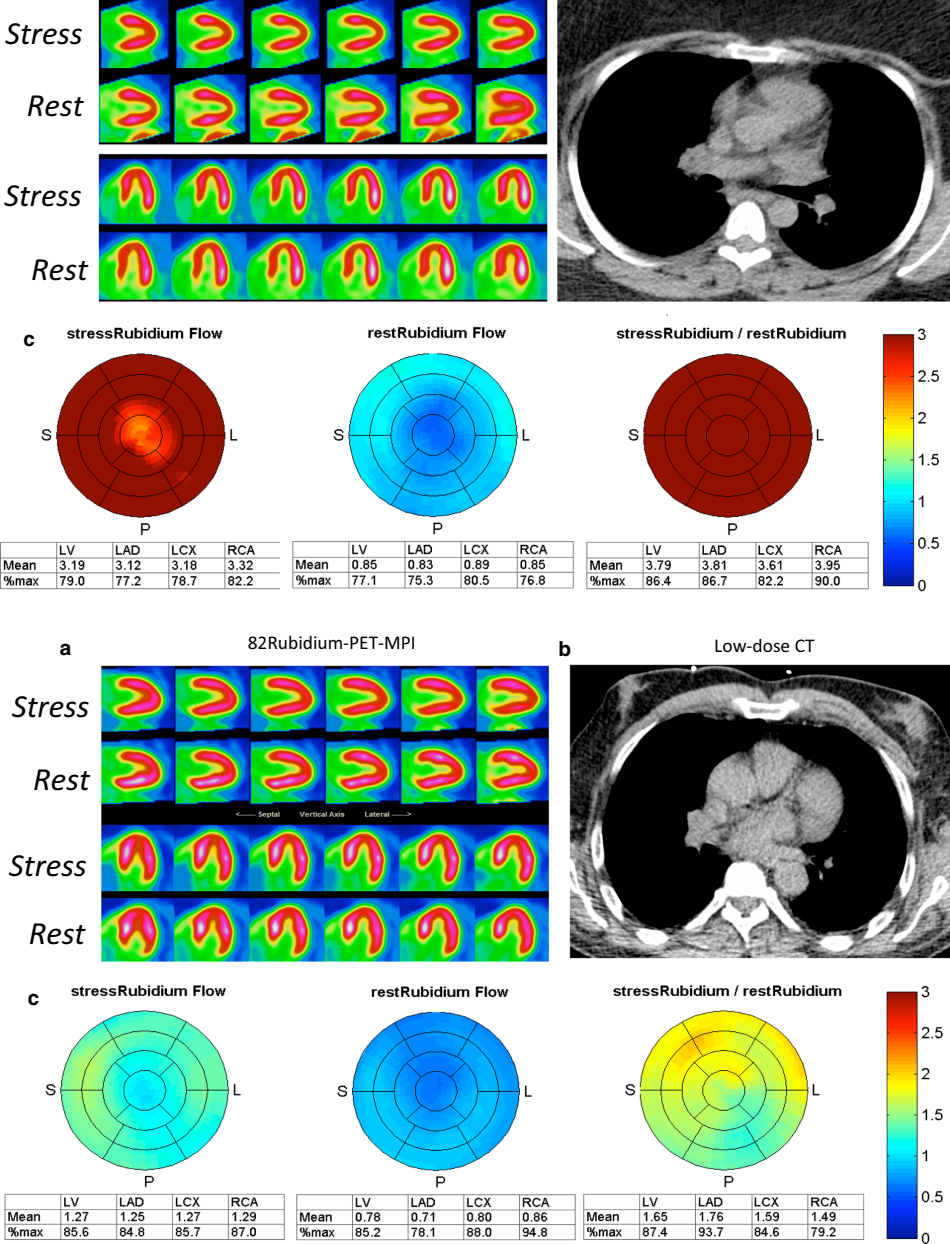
Representative examples of Rubidium-PET myocardial perfusion imaging (MPI) of diabetic patients with normoalbuminuria (A) and macroalbuminuria (B). No myocardial ischemia was present on Rb-PET MPI (a), nor coronary calcification on the low-dose CT used for attenuation correction of PET images (b) in both patients. Quantification of myocardial blood flow (MBF) with Rb-PET evidenced the presence of a global normal stress MBF and myocardial flow reserve in favor of a normal cardiac microvascular function (MFR = 5.1) in normoalbuminuric patient and a global low stress MBF and myocardial flow reserve in favor of cardiac microvascular dysfunction (MFR = 1.6) in macroalbuminuric patient (c)
